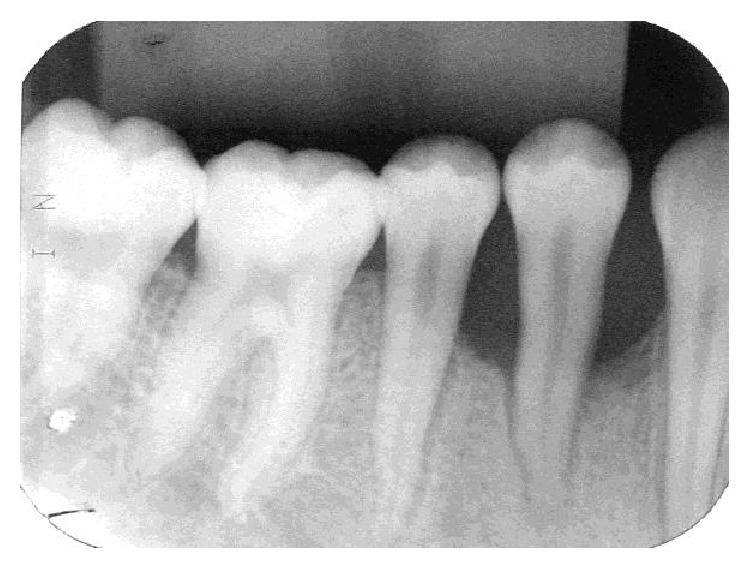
The radiographic image, where we can observe the extensive horizontal bone loss.
